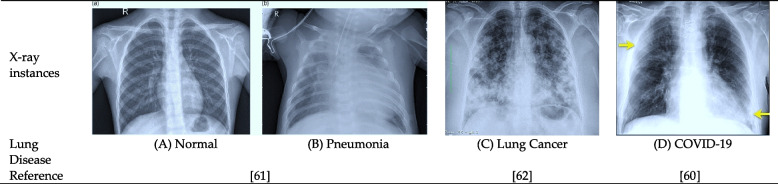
Instances of chest X-ray for prominent lung diseases
